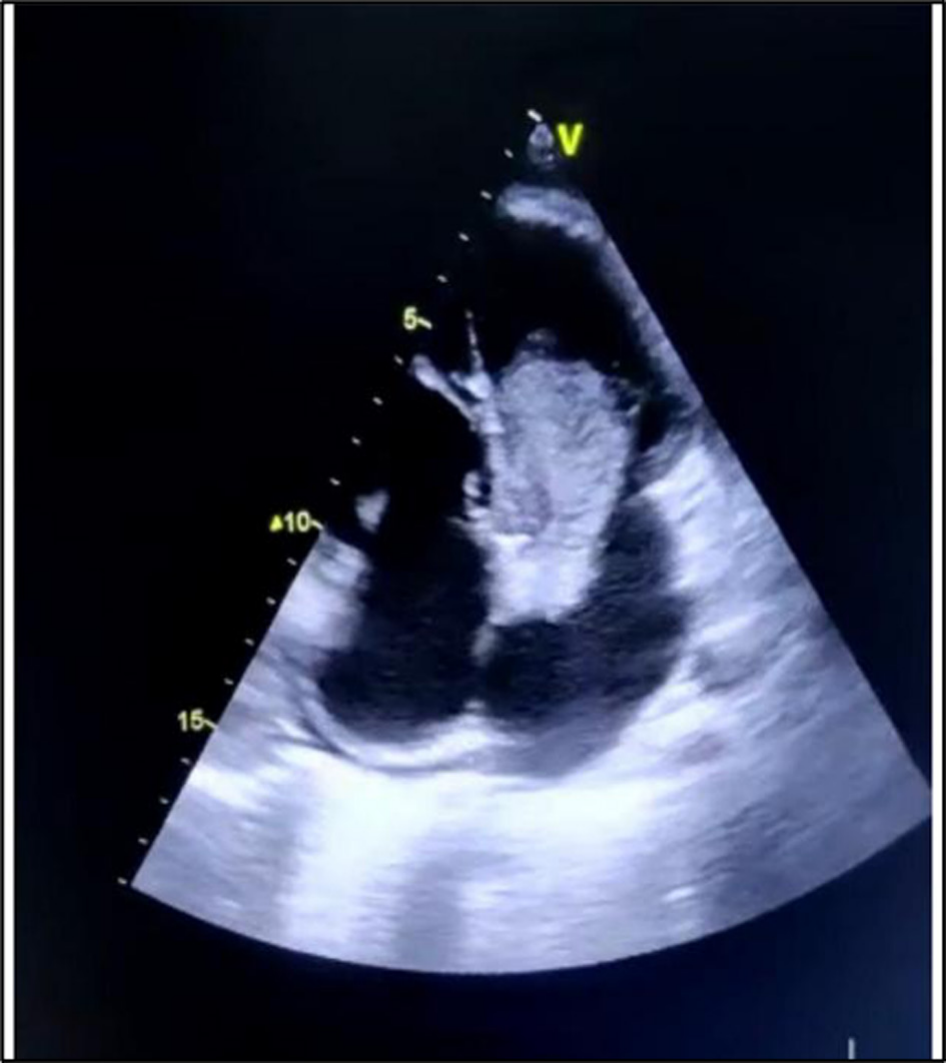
2D echocardiogram apical 4‐chamber view showing 39 × 20 mm left atrial myxoma protruding into the left ventricle across the mitral valve.
